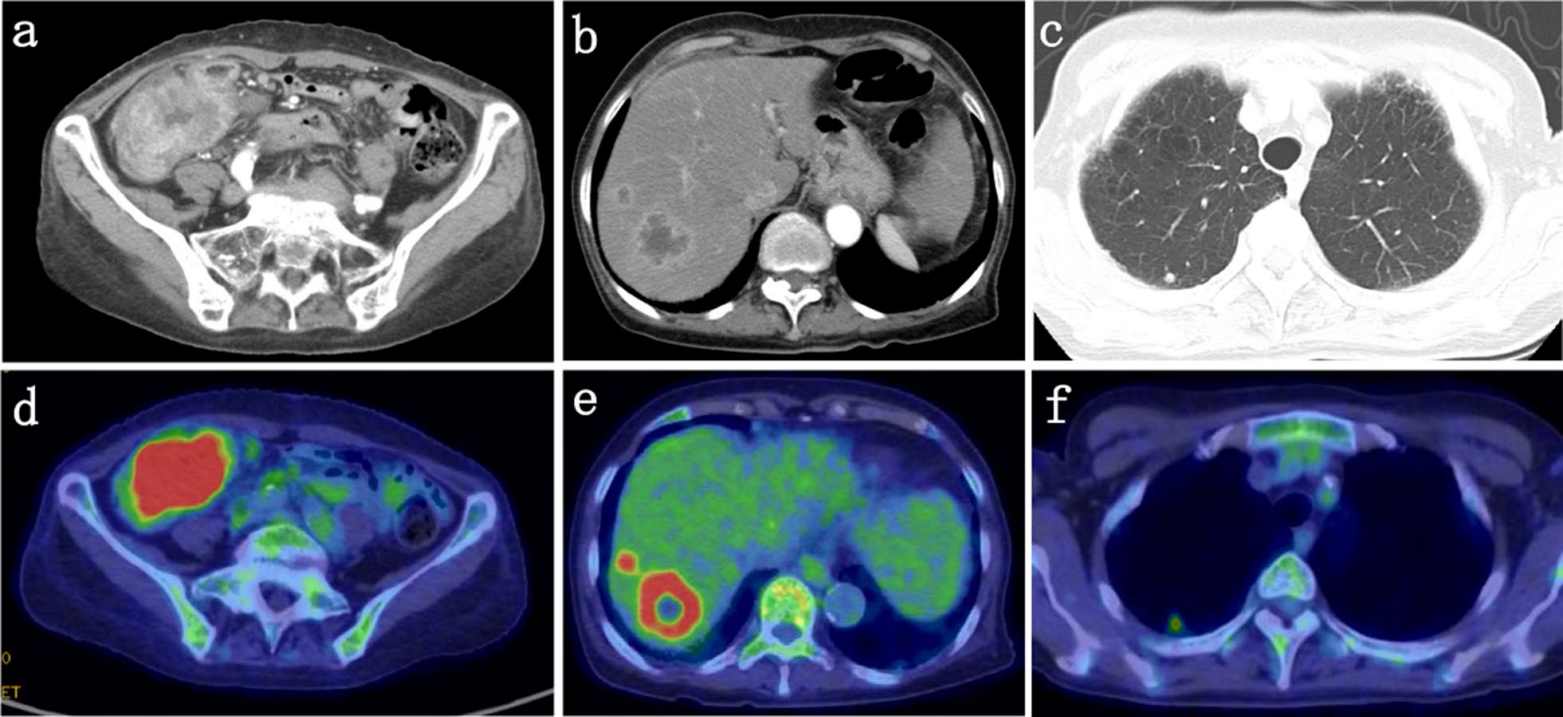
CT scan showed thickening of the cecum wall (a) and multiple masses in the liver (b), a single nodule in right lung (c). FDG-PET/CT revealed elevated uptake in the cecum and masses in the liver and a right lung with a standardized uptake value of 23.7 (d) and 8.6/5.9 (e), 4.9 (f), respectively. CT = computed tomography. FDG-PET/CT = 18F-fluorodeoxyglucose-positron emission tomography/computed tomography
